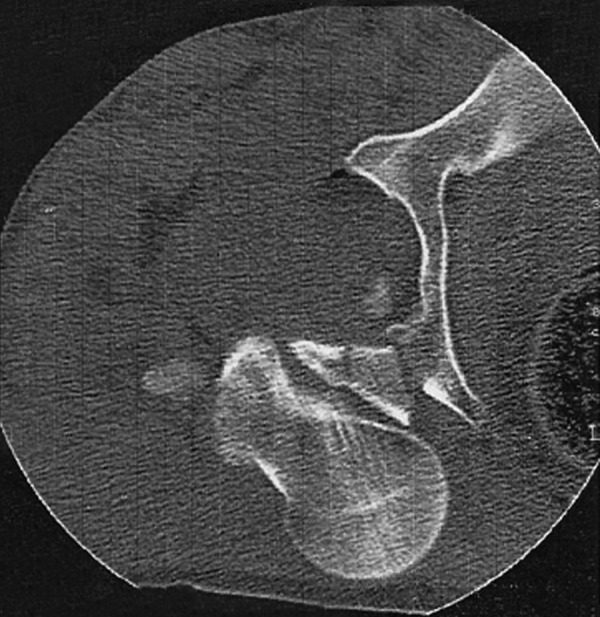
Computed Tomogram on Admission
